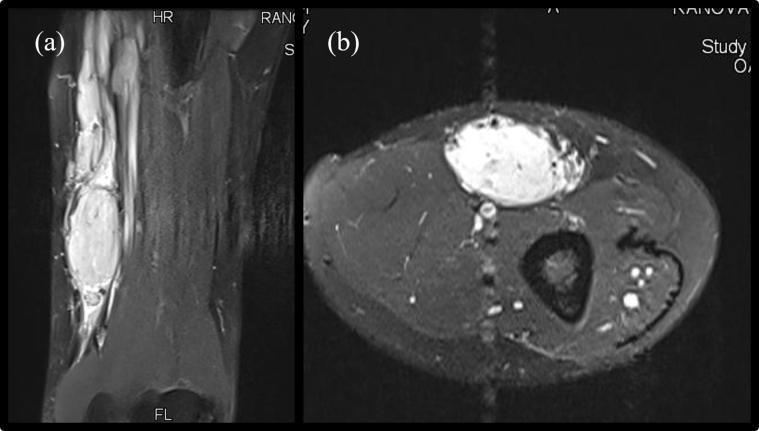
MRI of the upper arm showing a spindle shaped contrast enhancing mass in the median nerve course. (a) coronar view (b) axial view.
